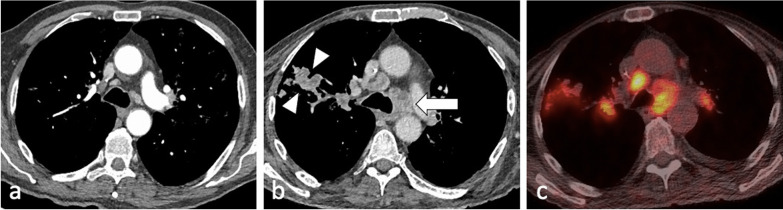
A 74-year-old male with HIV and a CD4 count of 10 cells/mm^3^ was started on antiretroviral therapy. He developed immune reconstitution inflammatory syndrome with worsening imaging findings in keeping with paradoxical reaction. Compared to the CT from three months prior (mediastinal window, axial plane—a), there are enlarged centrally necrotic precarinal lymph nodes (mediastinal window, axial plane—b, arrow) and necrotic nodules in the right upper lobe (arrowheads). These were avid on PET-CT (c)
